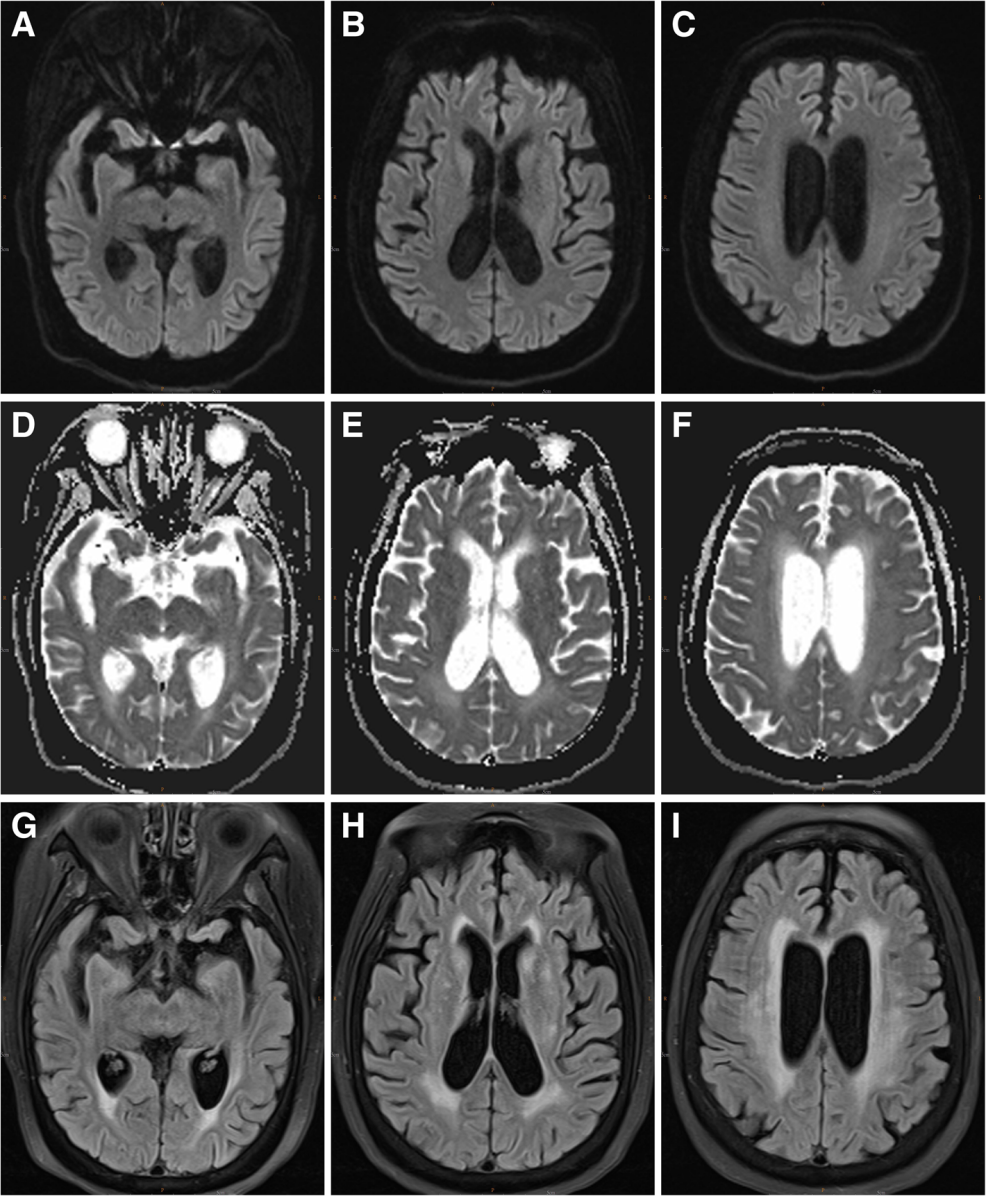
Repeat axial magnetic resonance imaging conducted 1 month after the patient received sliding-scale insulin therapy and rehabilitation. Axial diffusion-weighted imaging (a, b, c), apparent diffusion coefficients map (d, e, f), and T2-FLAIR imaging (g, h, i) revealed disappearance of the lesions along the corticospinal tract, from the levels of the cerebral peduncle (a, d, g) and the posterior limbs of the internal capsule (b, e, h) to the level of the corona radiata (c, f, i), possibly owing to the preservation of motor axons by the presence of the intramyelinic clefts
